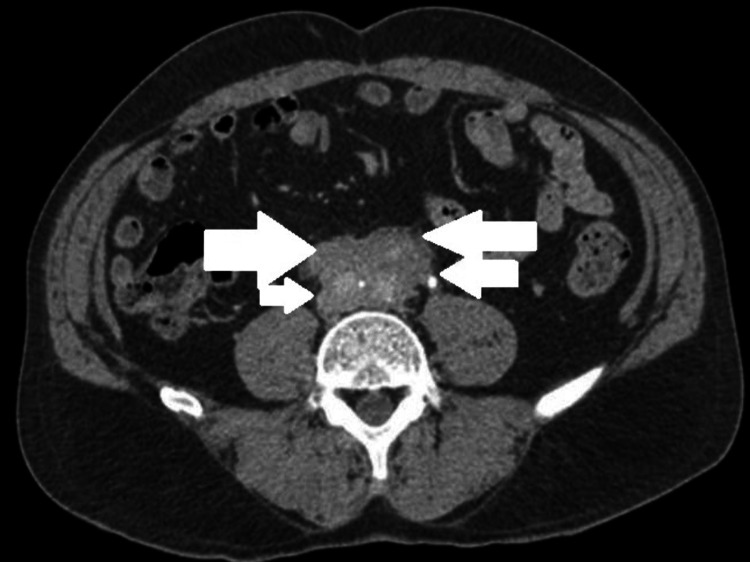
MDCT abdomen and pelvis showing an enhancing soft tissue density mass around abdominal aorta and bilateral common iliac arteries suggestive of idiopathic retroperitoneal fibrosis (IRPF). MDCT - Multi-Detector Computed Tomography
